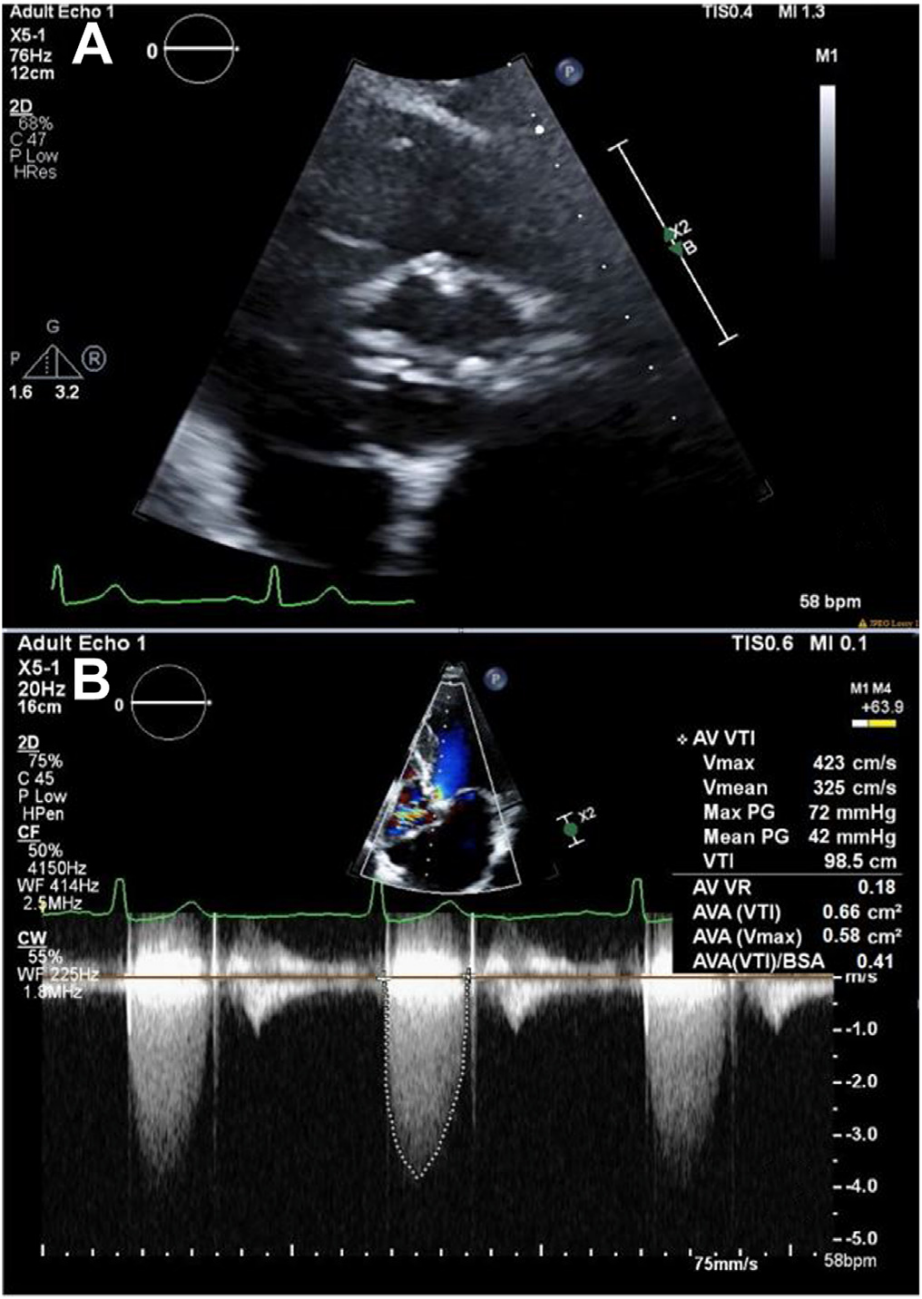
Transthoracic echocardiogramof the aortic valve and continuous wave transaortic valve doppler. (A) A short-axis TTE view of the bicuspid aortic valve. (B) TTE with Doppler analysis reveals an aortic valve peak velocity of 4.23 m/s and a peak transaortic PG of 72 mm Hg, mean transaortic PG of 42 mm Hg, and a calculated aortic valve area (AVA) of 0.66 cm^2^, corresponding to an AVA-to-body mass index of 0.41 cm^2^/m^2^.
